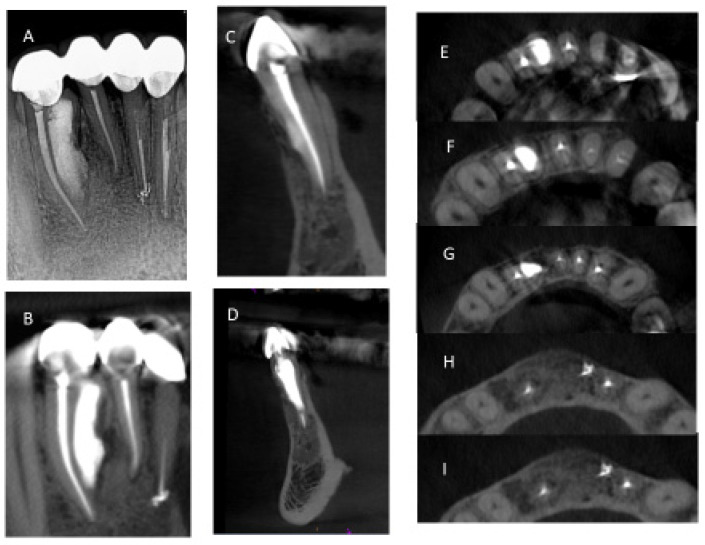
Post-treatment CBCT at 36 month follow-up showed bony healing on 2D image (A) and on small FOV CBCT coronal section (B), sagittal section (C,D), and axial sections (E–I).
